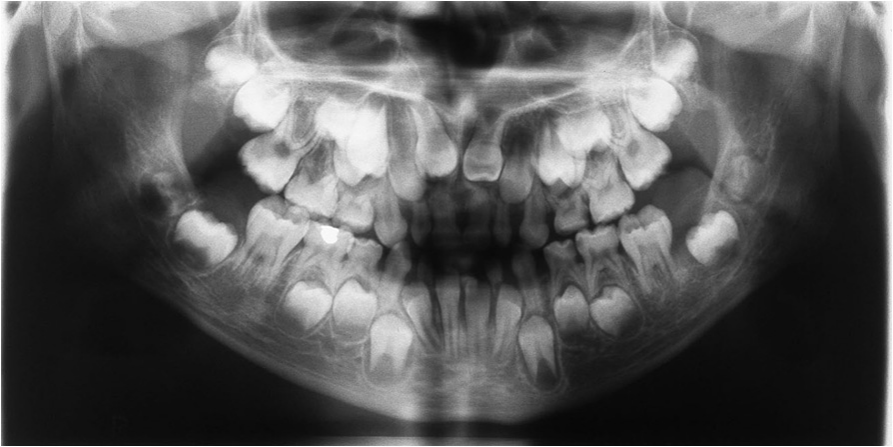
Panoramic X-ray showing supernumerary teeth and impaction of the upper anterior teeth. (From Kolokitha OE, Papadopoulou AK [1] with permission).
